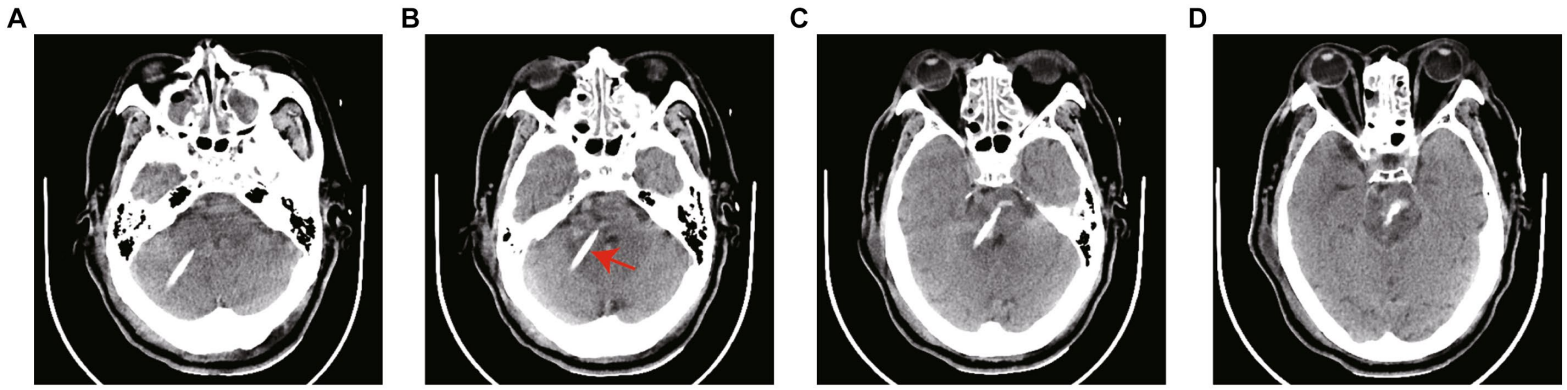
(A–D) CT scans 12 h after surgery. The arrow in the figure refers to the drainage tube. The hematoma was dissolved by injecting 20,000–30,000 IU of urokinase into the hematoma cavity once a day. The drainage tube was removed according to the results of CT scanning.
